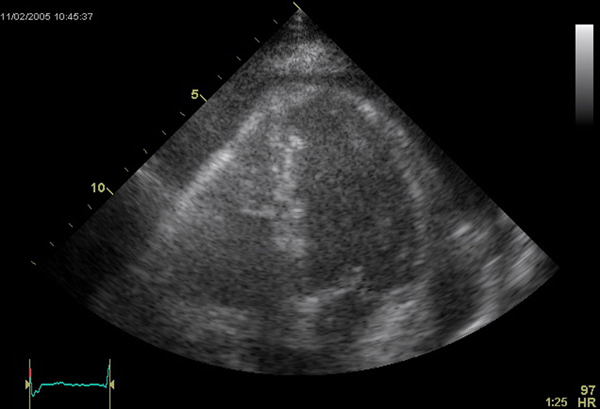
Transthoracic echocardiography (apical 4-chamber view) demonstrating a large pericardial effusion and a thickened pericardium of the free wall of the right ventricle (see SD1 and SD2).
